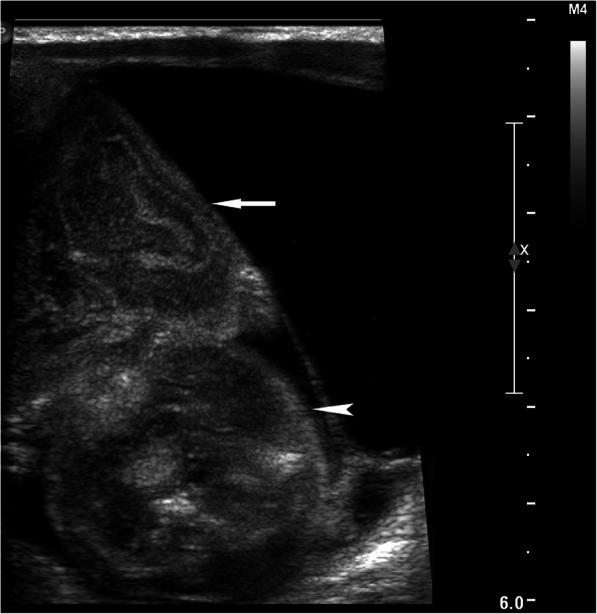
Transverse grayscale high-frequency ultrasound imaging: Ultrasound revealed a 5.5 cm × 5.0 cm heterogeneous, complex cystic and solid intraabdominal mass, which appears as a head (arrow) and body (arrowhead) in shape
